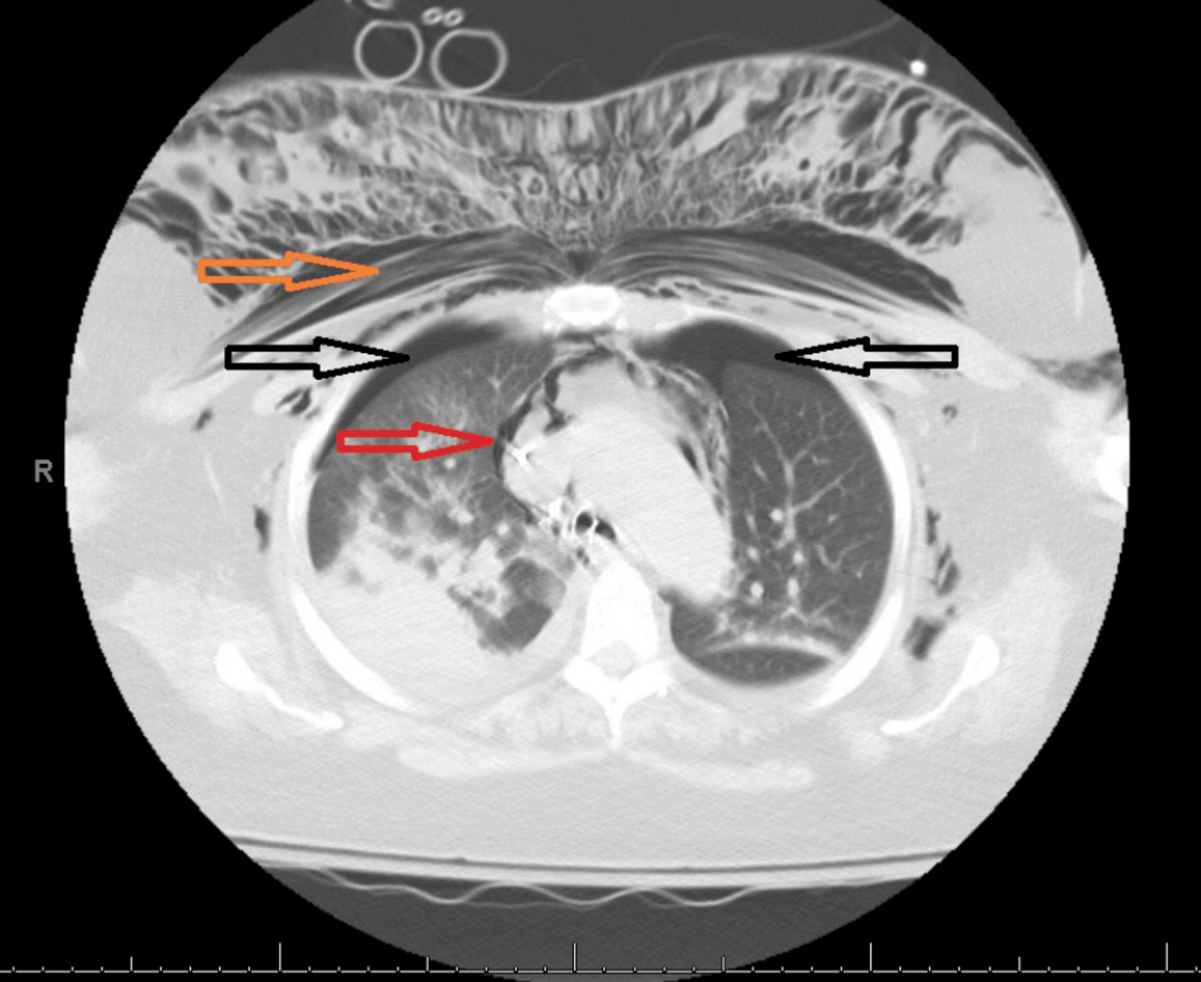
CT chest showing bilateral pneumothroaces (black arrows), pneumomediastinum (red arrow), and subcutaneous emphysema (orange arrow)
